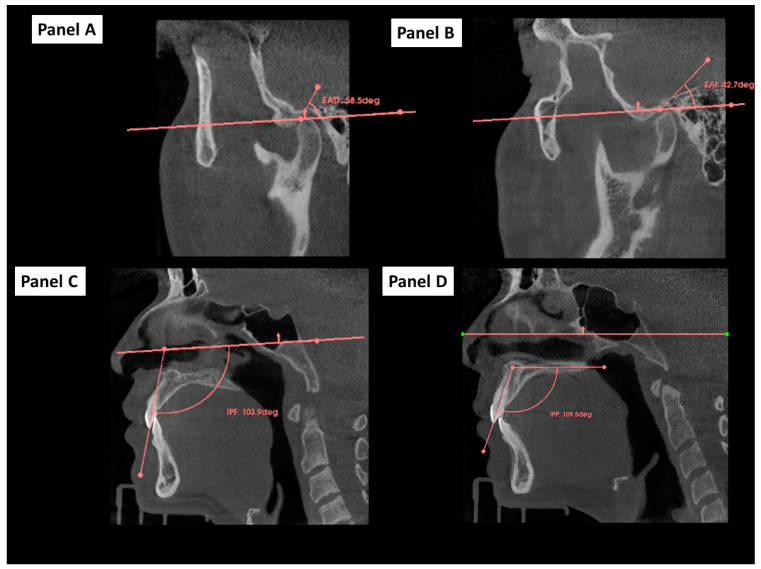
Determination and measurements of 3D angles in CBCT using 3D Slicer Software. Footnote: Panel (A): measurement of the left articular eminence angle (EAD). Panel (B): measurement of the right articular eminence angle (EAD). Panel (C): measurement of the upper incisor angle relative to the Frankfort plane. Panel (D): measurement of the upper incisor angle relative to the palatal plane.
